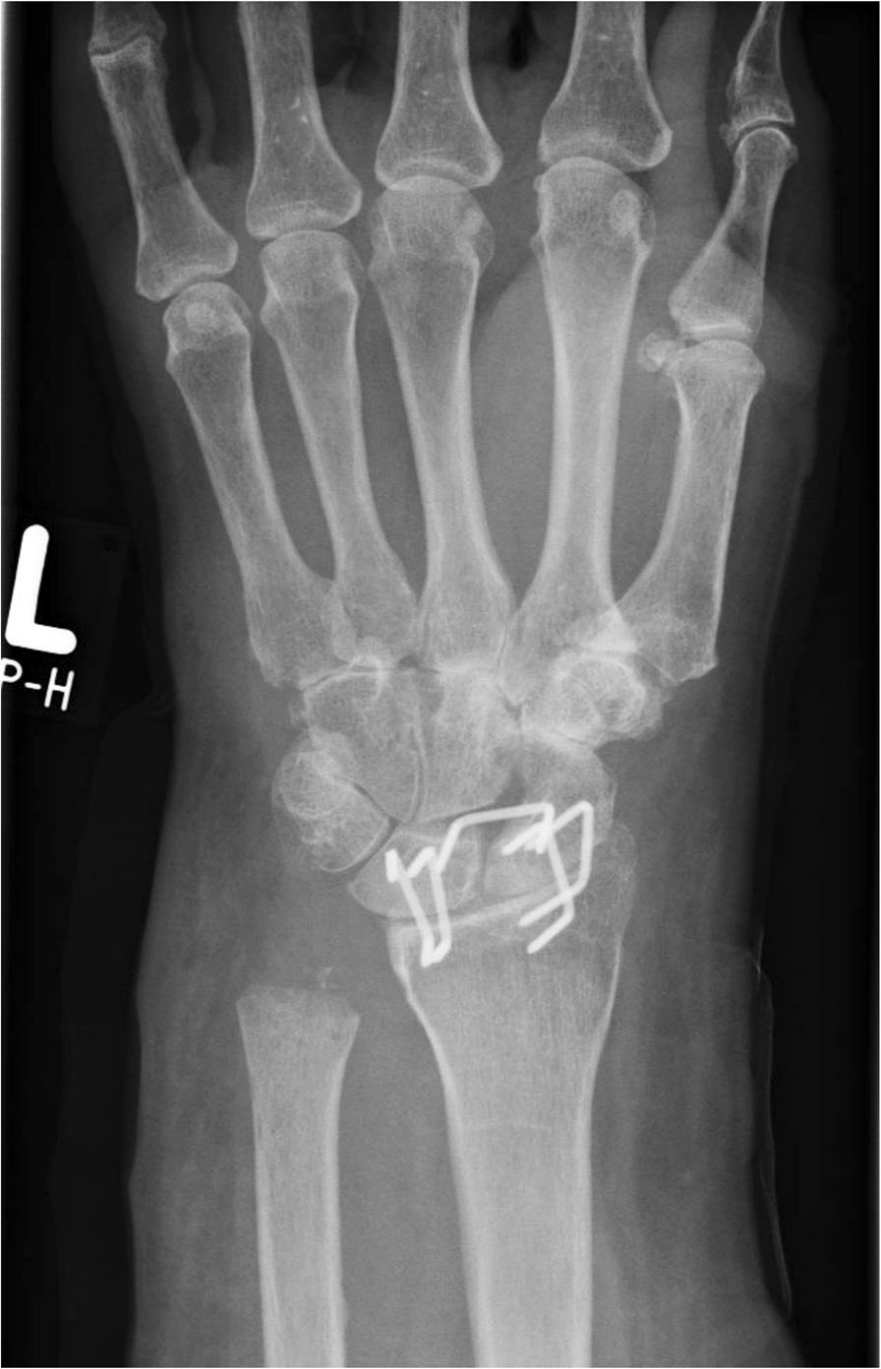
Postoperative X-ray of the wrist after proximal fusion and distal RIAP
